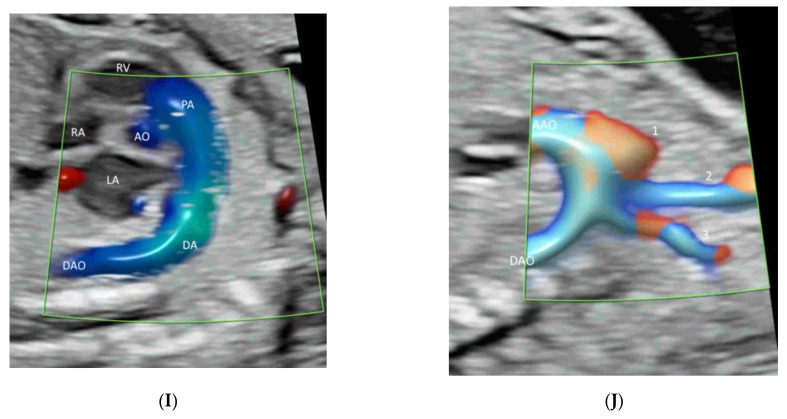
Color flow imaging in fetal echocardiography: Ductus venosus (DV) and inferior vena cava (IVC) (A), pulmonary veins (PV), atrial septum and foramen ovale (B), atrioventricular valves (C), ventricular septum (D), semilunar valves (E–H), ductal arch (I), and (g) aortic arch (J). Note the left ventricular outflow tract (LOT) in (E), branches of RPA in (F), three-vessel view in (G), three-vessel trachea view in (H), and the three ascending branches (1–3) in (J). LPV, left portal vein; LHV, left hepatic vein; RA, right atrium, LA, left atrium; RV, right ventricle; LV, left ventricle; IAS, interatrial septum; IVS, interventricular septum; DA, ductus arteriosus; PA, pulmonary artery; RPA, right pulmonary artery; Tr, trachea, AZ, azygous vein; AO, aorta; DAO, descending aorta; AAO, ascending aorta.
